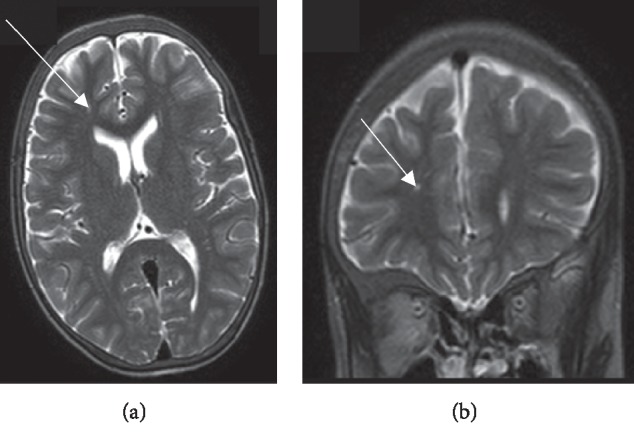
(a) Axial T2-weighted MRI brain scan demonstrating focal hyperintensity (arrow), probably representing an infarctive lesion on the right frontal lobe deep white matter. (b) The same lesion as seen on the coronal T2-weighted MRI brain scan.
